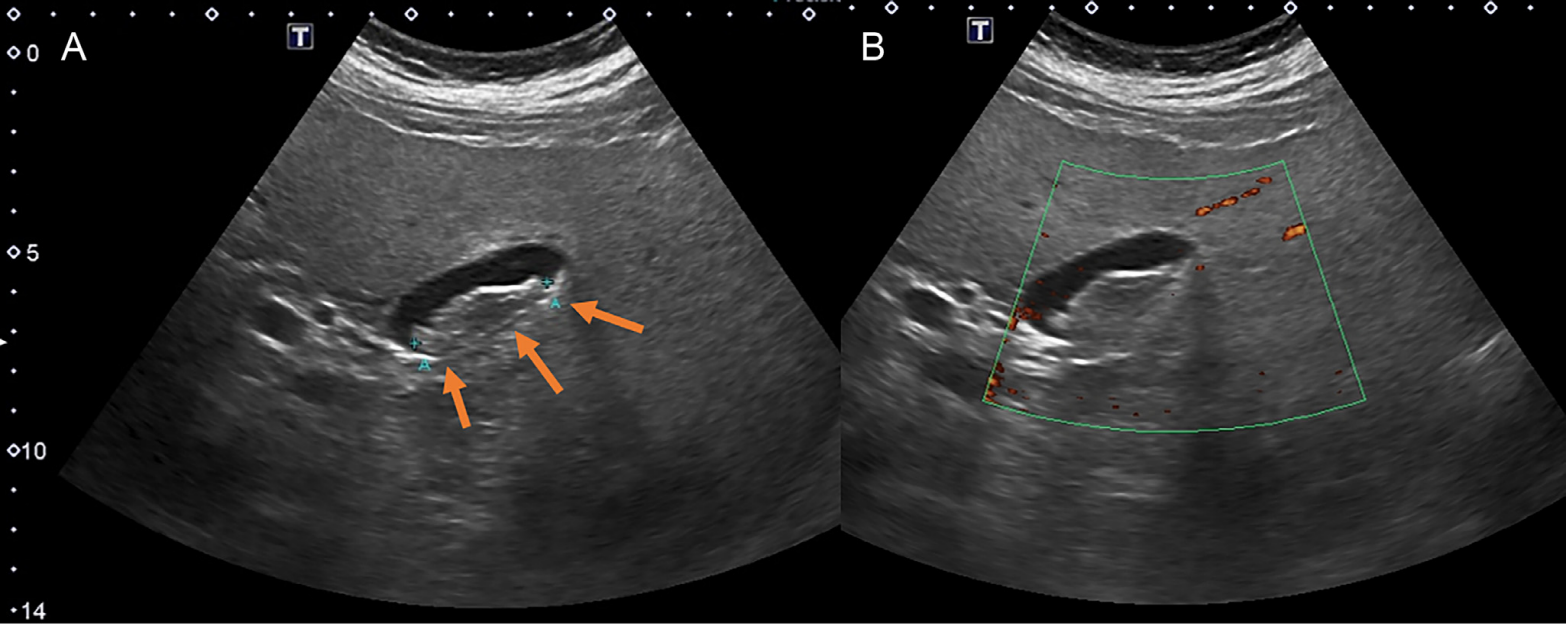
(A) Transverse grayscale ultrasound image of the gallbladder demonstrates a 3.7 cm well-circumscribed intraluminal lesion (arrows) which is isoechoic to liver parenchyma. (B) On power Doppler assessment, there is no vascularity demonstrated within the gallbladder lesion.
